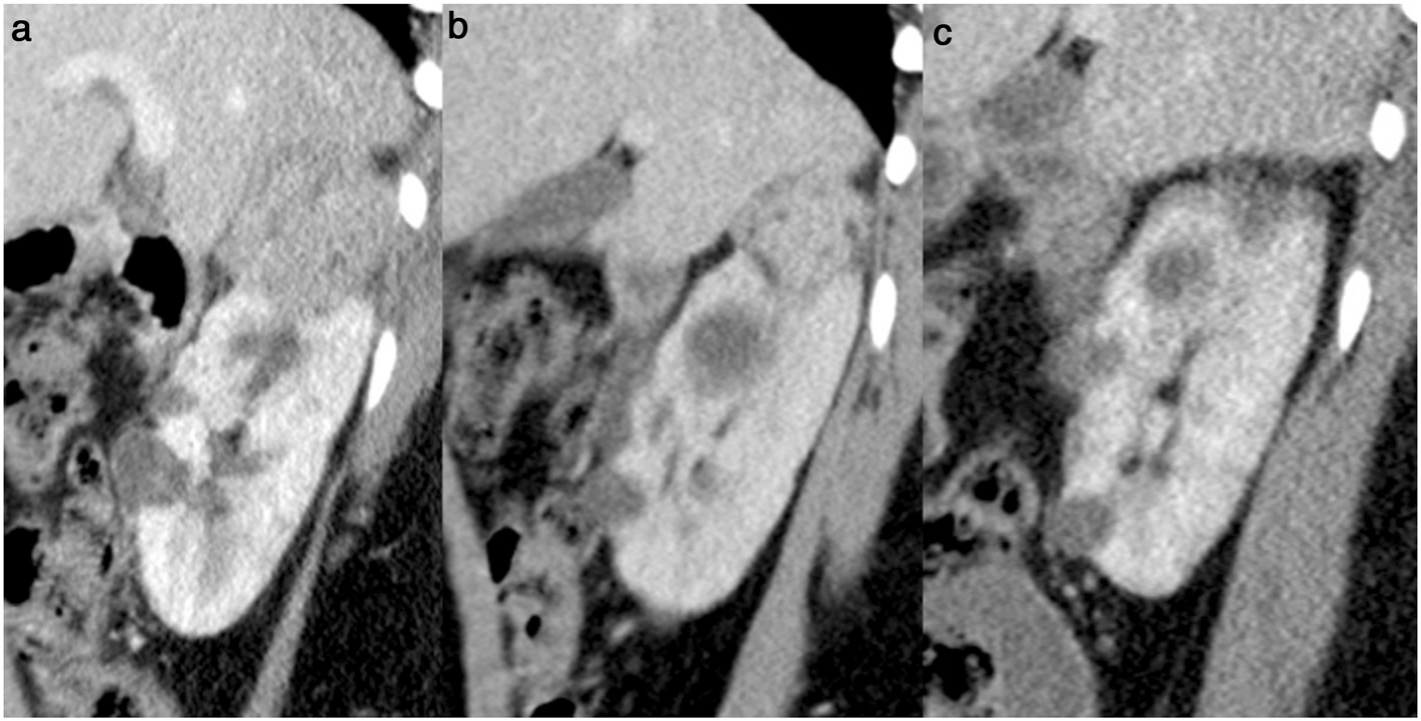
Sagittal contrast enhanced (portal phase) computed tomography scan of the right kidney showing the angiomyolipoma of the upper pole. (a) before treatment (left image); (b) after 12 months of treatment with sirolimus (middle image), showing a decrease in tumor size; and (c) one year after cryoablation (right image) showing devascularisation and shrinkage of the tumor, consistent with a complete ablation.
